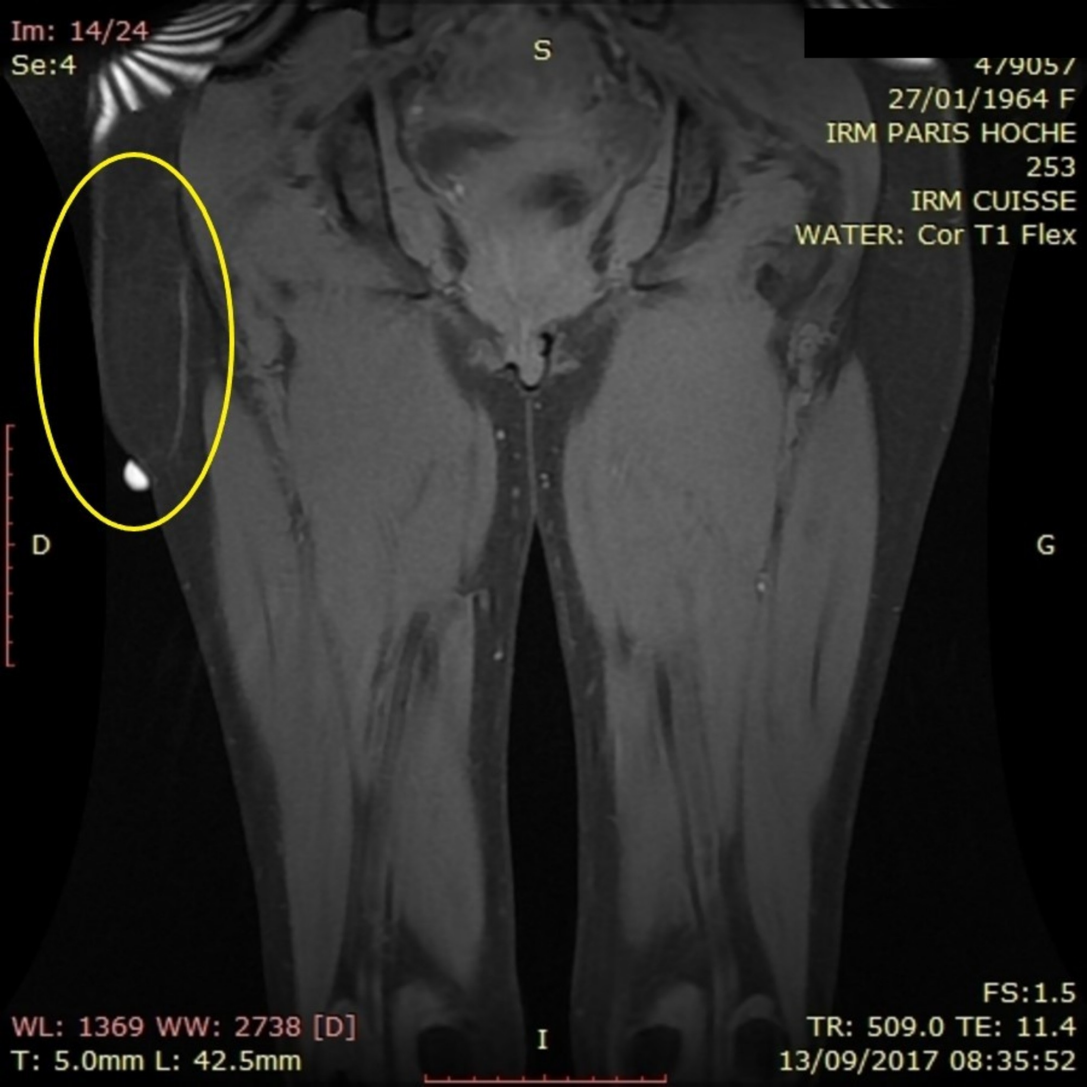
Coronal view of a lower extremity MRI showing the pseudolipoma in the yellow circle. MRI: Magnetic resonance imaging
